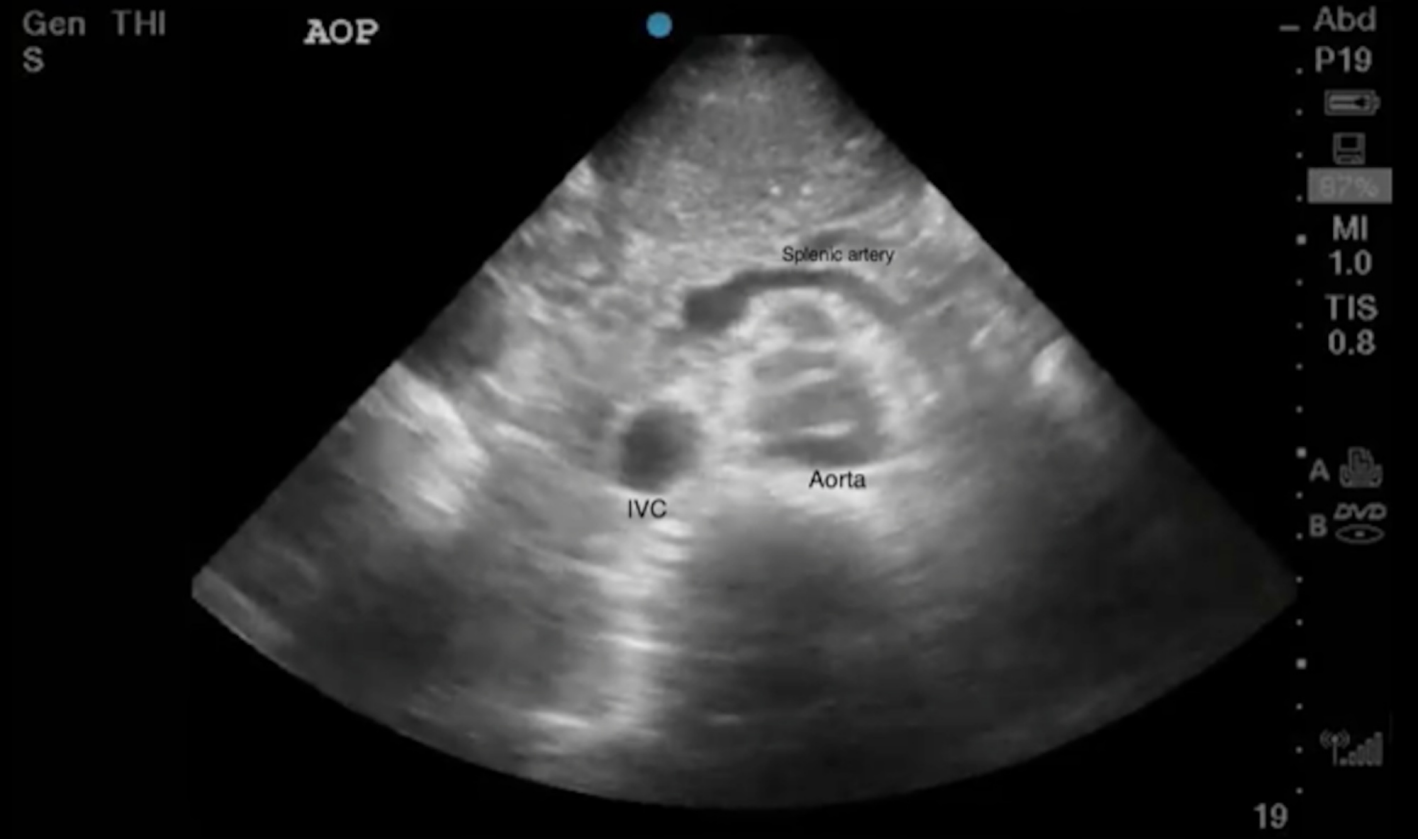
Transverse ultrasound image of intimal flaps in the aorta from a dissection
